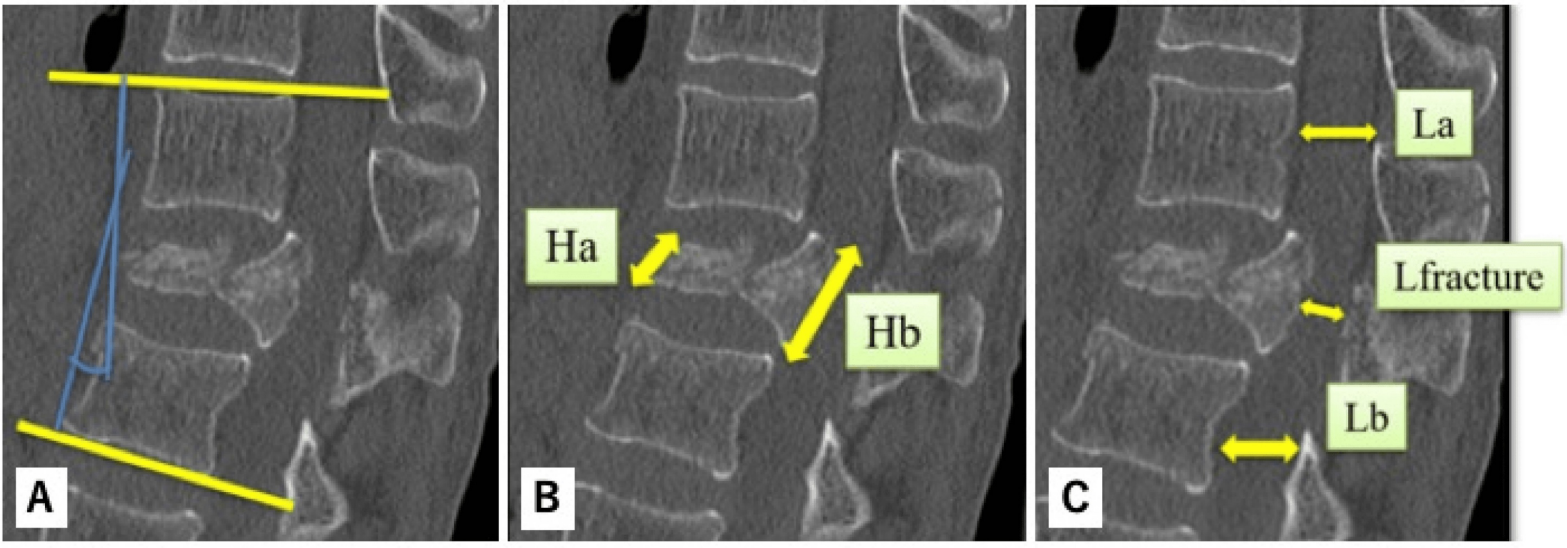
Radiological assessments using computed tomography (CT). (A) Local kyphosis angle. (B) Wedge deformity rate. (C) Spinal canal stenosis rate. Ha: Height of the anterior wall of the fractured vertebra; Hb: Height of the posterior wall; Lfracture: anterior-posterior diameter of the spinal canal at the fracture site; La: diameter at the cephalad vertebra; and Lb: diameter at the caudal vertebra. The image is created by the author (Shunsuke Kobayashi) of this study.
